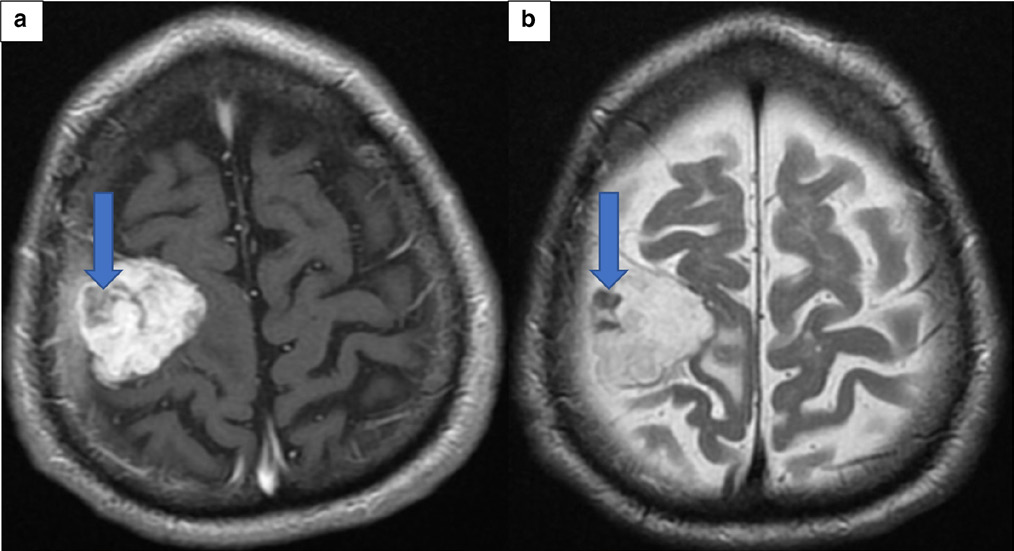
(A) Post-Contrast T1 Axial MRI Brain. (B) Post-Contrast T2 Axial MRI Brain. These demonstrate an area which does not enhance within the meningioma on both T1- and T2-weighted images (Blue Arrow). This area represented the metastatic deposit
